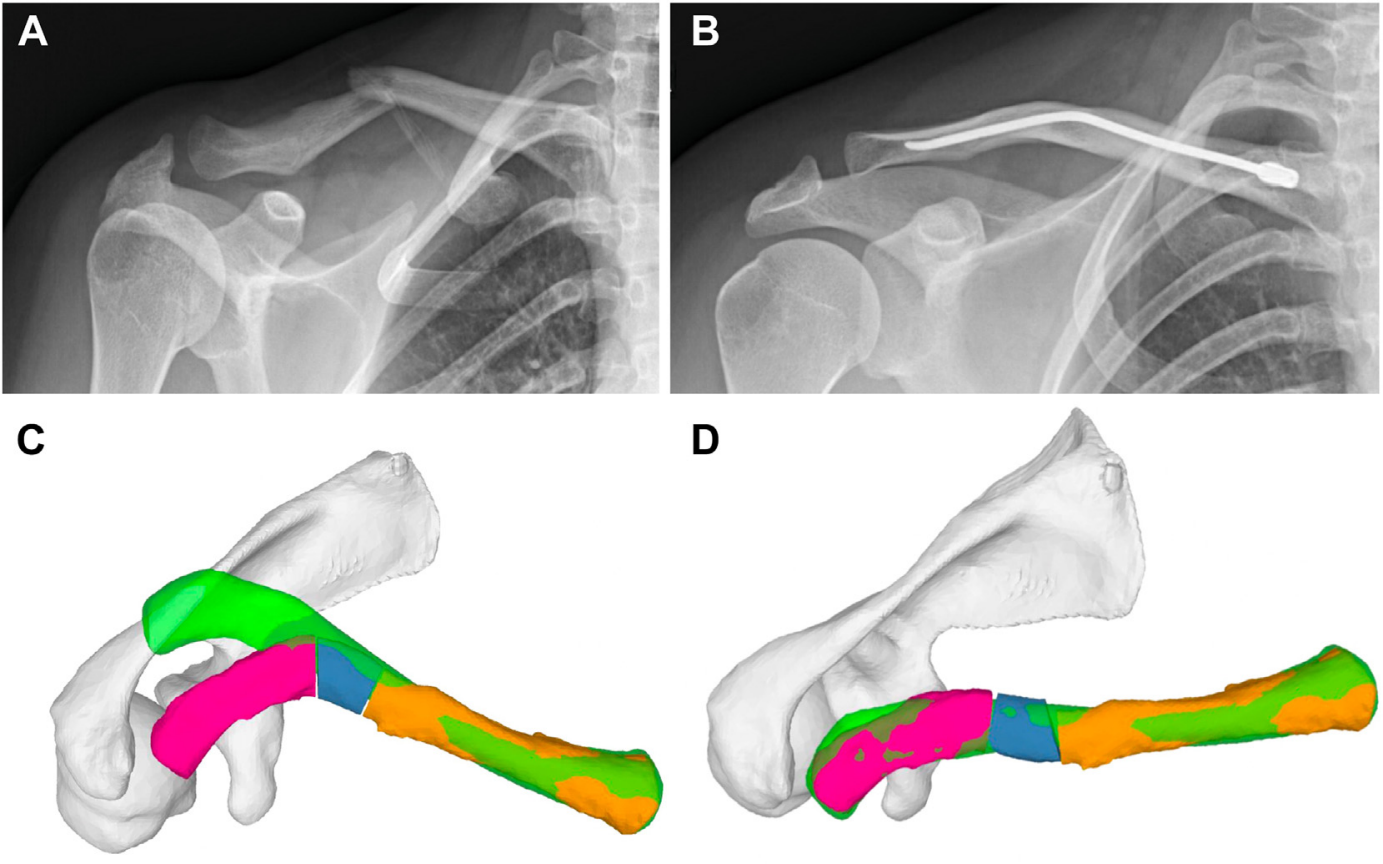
This is a case of a 35-year-old patient with a malunited mid-shaft clavicle fracture after ORIF with a nail (A and B). The reconstructed 3D models of the patient’s segmented CT data of both clavicles were imported into planning software. The model of the contralateral anatomy (clavicle and scapula) was mirrored and used as a reconstruction template (C and D).
